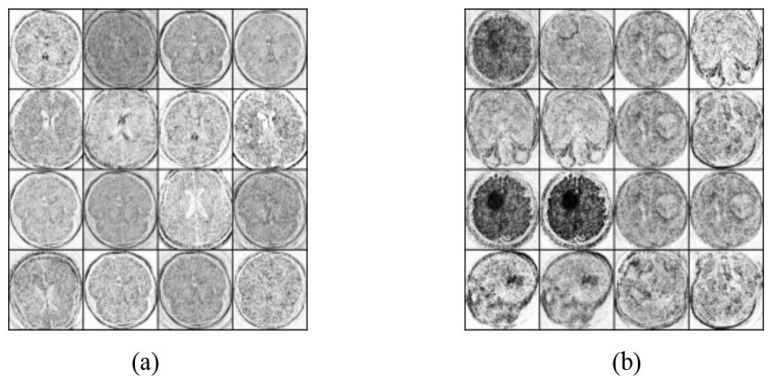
MRI scan images that are generated by applying Vanilla GANs for (a) Vanilla GANs no tumor images, and (b) Vanilla GANs tumor images.
